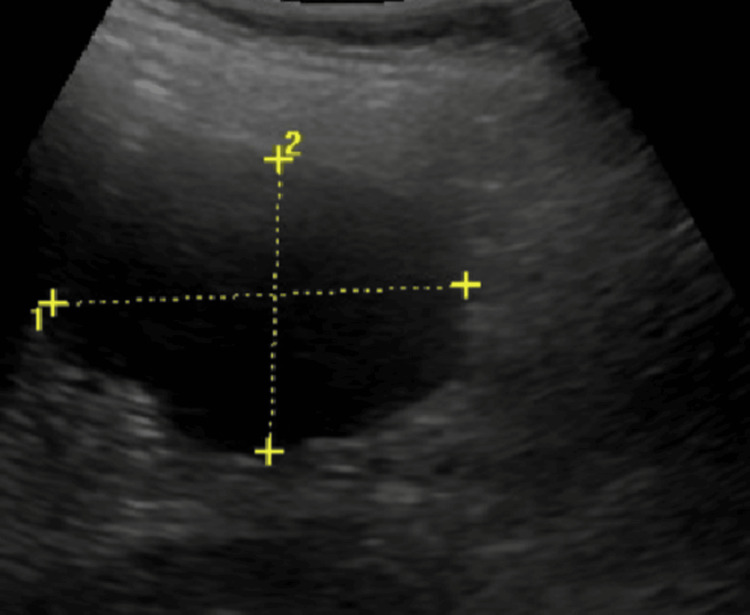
Repeat ultrasound of the abdomen The five-day follow-up ultrasound showed no significant interval change (5.0 × 3.5 cm).
